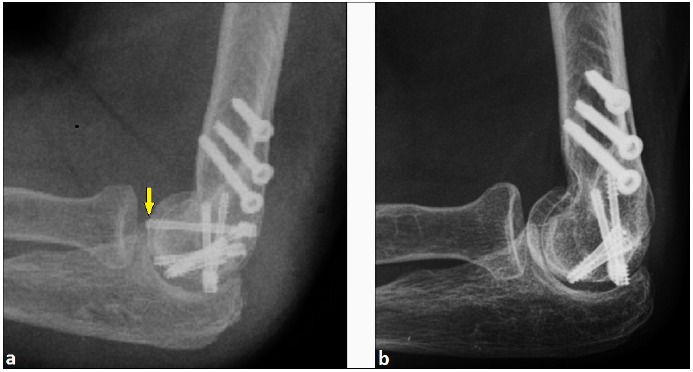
(Case Presentation): (a) Lateral radiograph three years after ORIF showing migration of one of the headless compression screws into radiocapitellar joint (arrow) despite complete union of CSF. (b) Lateral radiograph after removal of all three headless compression screws of capitellum showing no signs of posttraumatic/postoperative OA.
